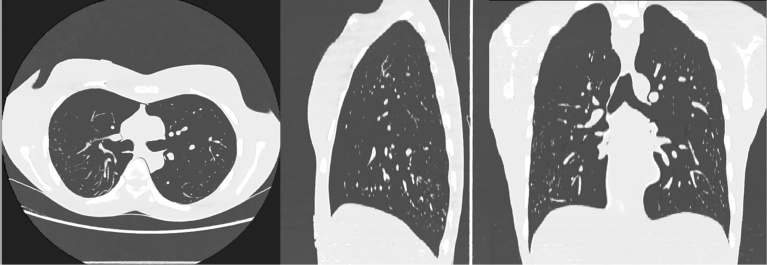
Axial, sagittal, and coronal images of the testing ULDCT which were obtained by denoising with CAE slice-by-slice. Abbreviation: ULDCT, ultra-low-dose CT; CAE, convolutional auto-encoder.
